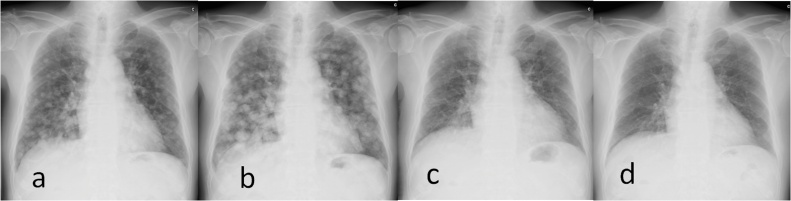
(a) Chest radiography showing innumerable lung nodules indicating metastatic disease 4 months after hepatectomy. (b) The follow-up chest radiography showing progression of the lung tumor 13 months after the first presentation. (c) The follow-up chest radiography showing spontaneous regression of the lung tumor 18 months after the first presentation. (d) Almost complete disappearance of the lung metastasis 41 months after the first presentation.
